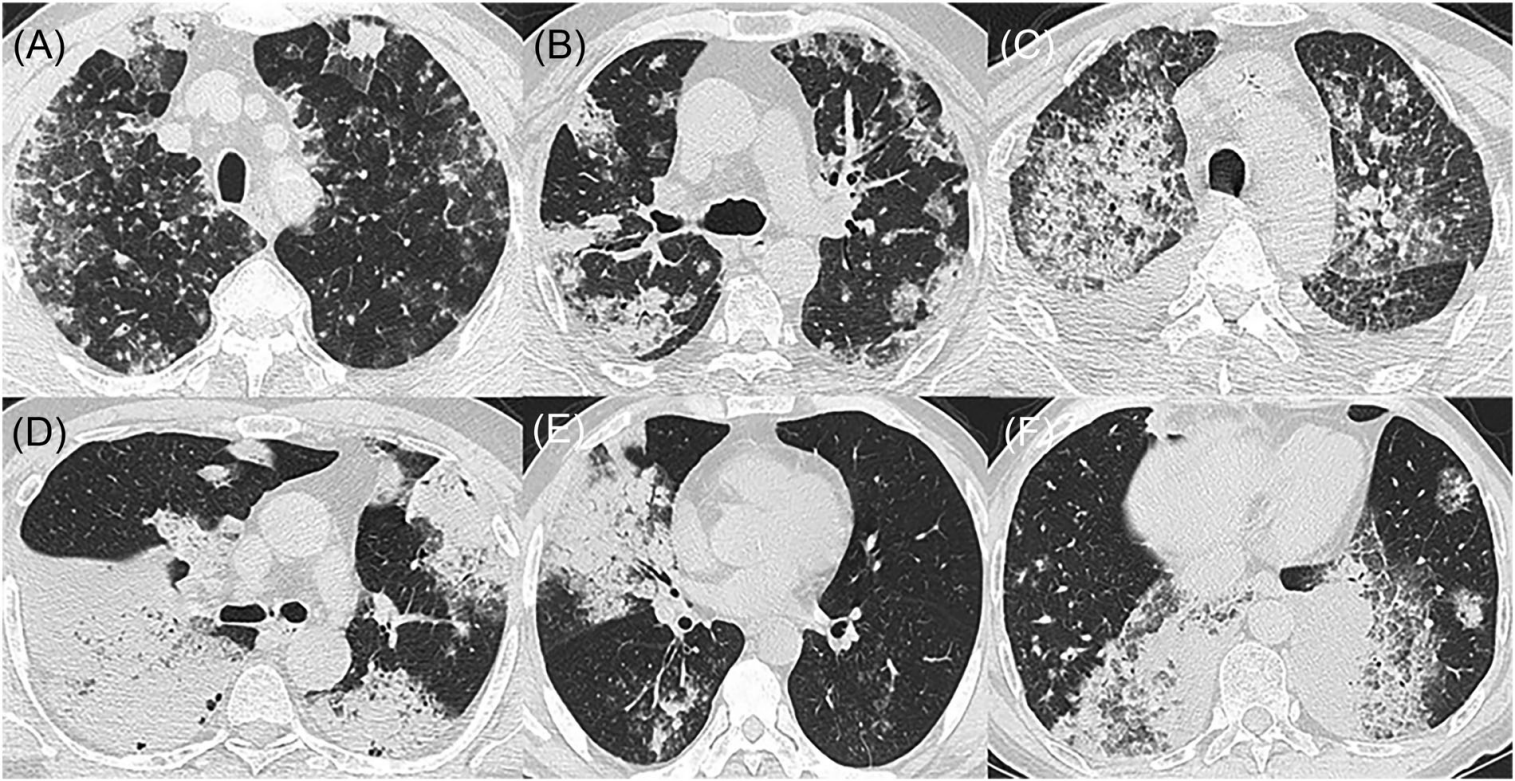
CT manifestations of pulmonary mucinous adenocarcinoma with diffuse pneumonia. (A) Patchy density was distributed in the upper lobe of both lungs. (B) Patchy density was distributed in the upper lobe of both lungs and the dorsal segment of the left lower lobe. (C) Patchy density was distributed in the upper lobe of both lungs and the dorsal segment of the left lower lobe, as well as bilateral pleural effusion. (D) Patchy density was distributed in the left and right middle lower lobes. (E) Patchy density was distributed in the right middle lobe and both lower lobes. (F) Patch density was distributed in the lower lobe of both lungs, mainly in the medial region, and in the lingual segment of the upper lobe of the left lung.
